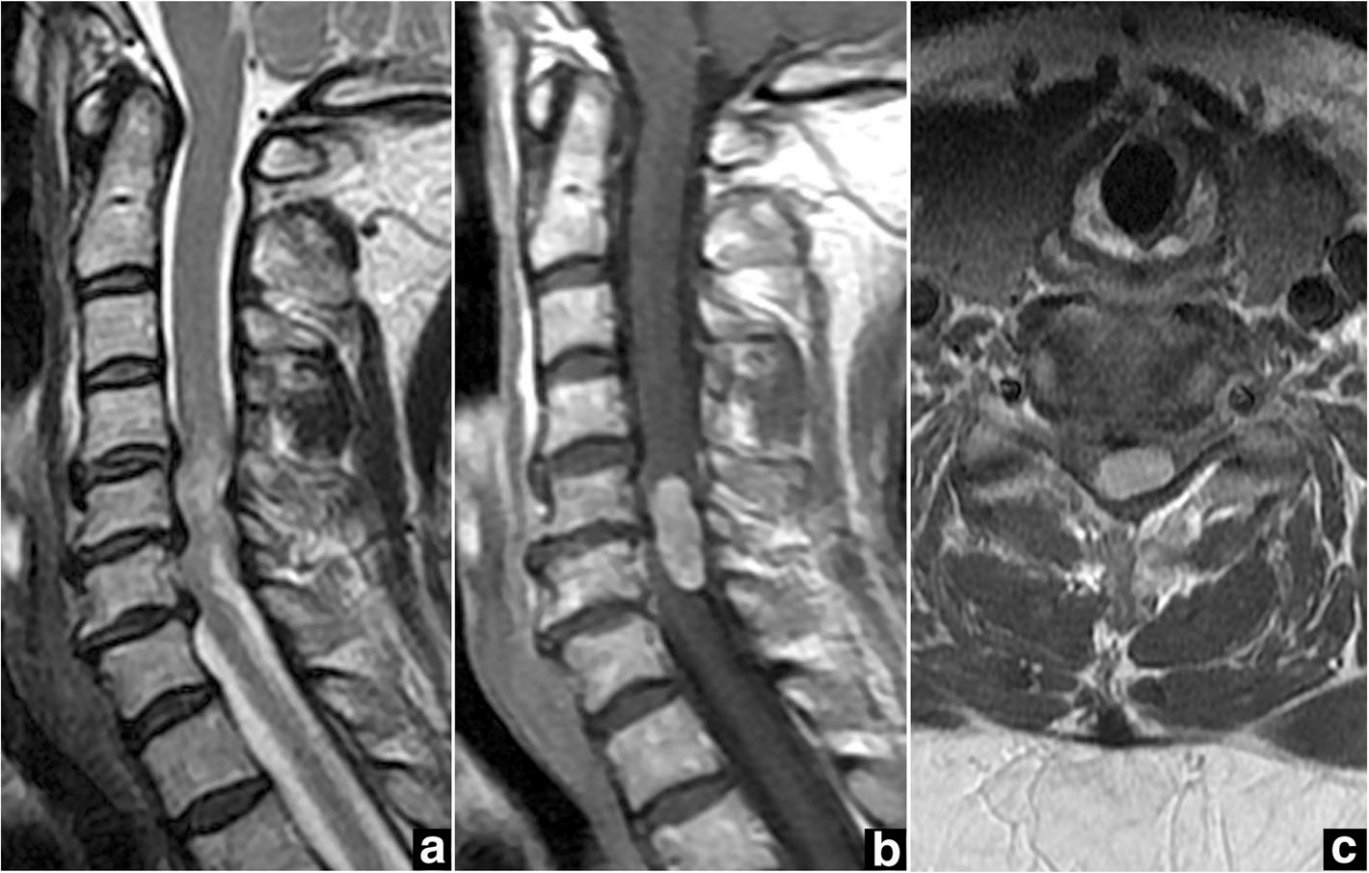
Case 2. Preoperative magnetic resonance imaging (MRI) of the cervical spine. a Sagittal T2-weighted MRI shows the intradural extramedullary spinal cord tumor with heterogeneous signal hyperintensity at C5-C6 and spinal canal stenosis at C4-C7. b, c Gadolinium-enhanced sagittal MRI (b) and axial MRI (c) at the C5-C6 level show homogeneous enhancement of a tumor located dorsally and to the left of the spinal cord
